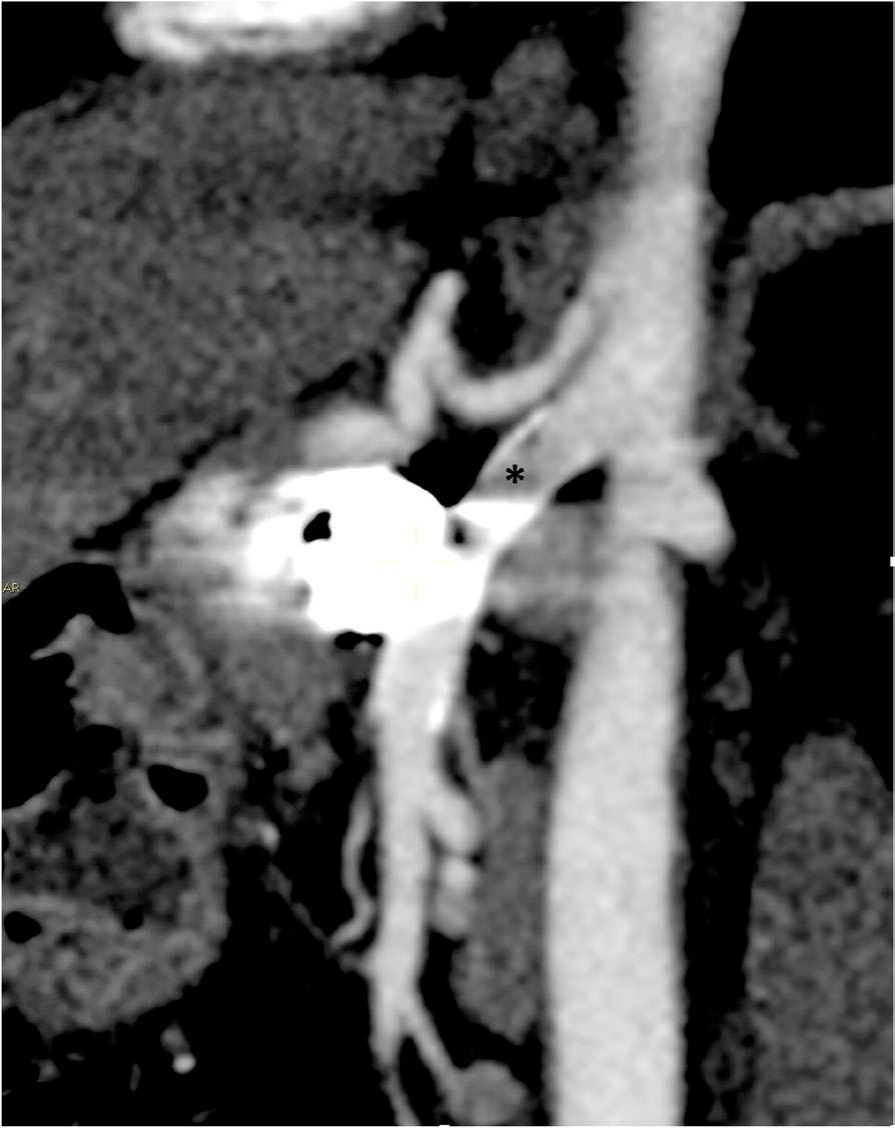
Contrast CT-scan sagittal reconstruction of SMA at 9 year follow-up showing the Viabahn patency. Imaging is noised by coils and orthopedic devices (*). Patient remains asymptomatic
